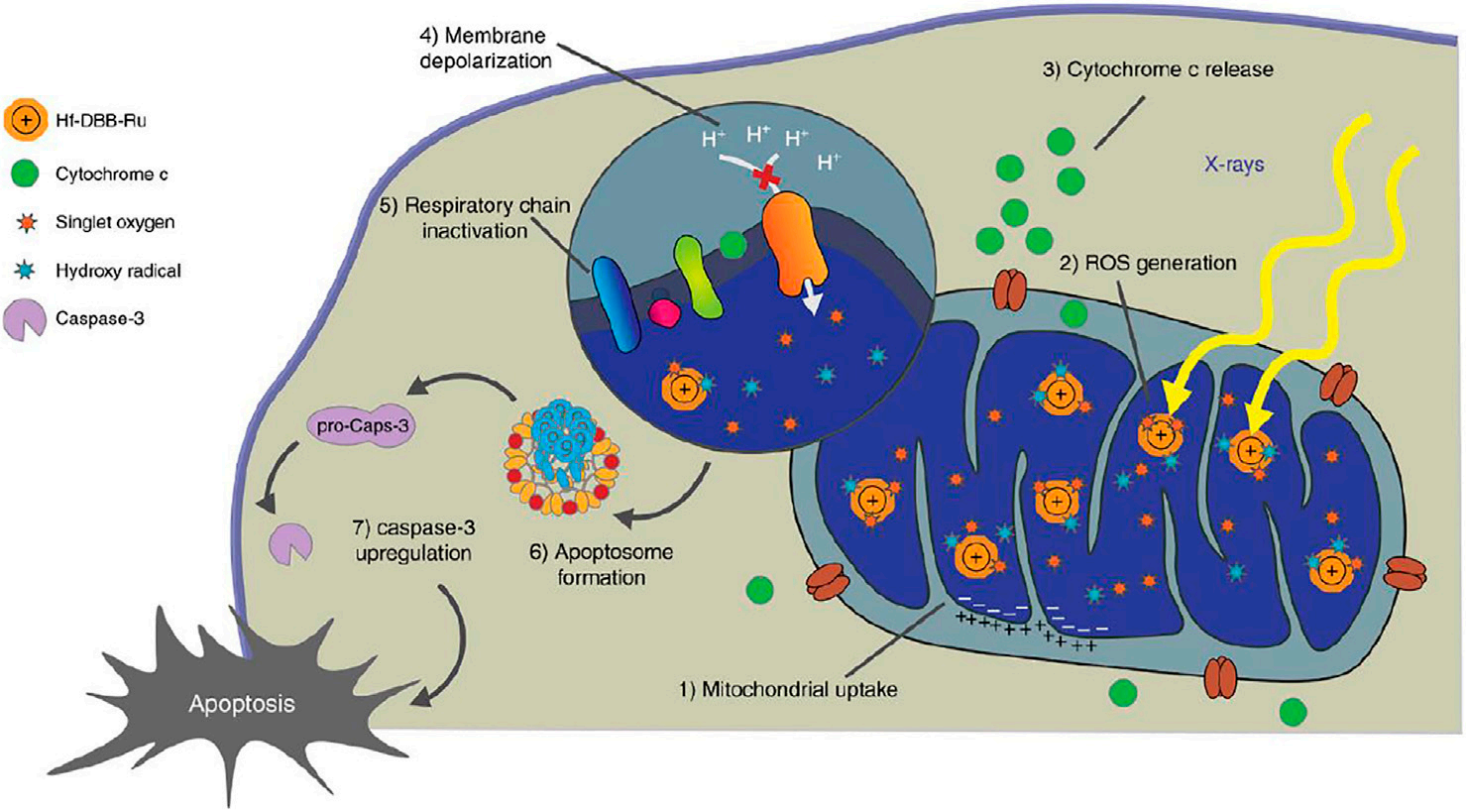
Mitochondria-targeted RT-RDT mediated by Hf-DBB-Ru. Hf-DBB-Ru was internalized by tumor cells efficiently and enriched in mitochondria due to dispersed cationic charges in the nMOF framework. Hf_6_ SBUs preferentially absorb X-rays over tissues to enhance RT by sensitizing hydroxyl radical generation and enable RDT by transferring energy to Ru(bpy)_3_
